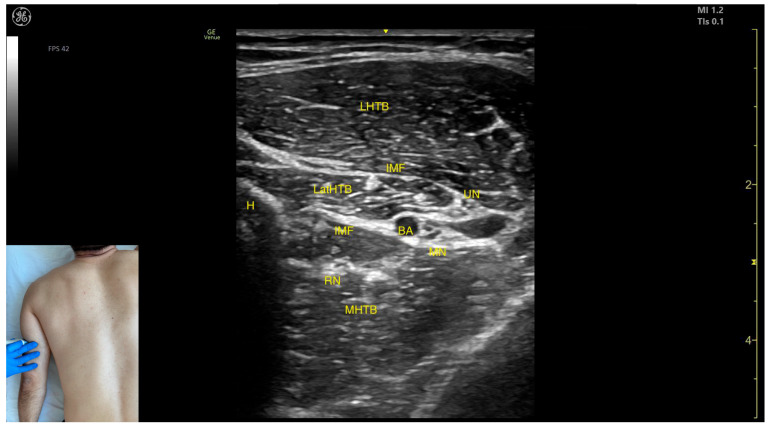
Ultrasound anatomy of the triceps brachii (TB) with key landmarks: LHTB—long head triceps brachii; IMF—intramuscular fascia; LatHTB—lateral head triceps brachii; MHTB—medial head triceps brachii; UN—ulnar nerve; RN—radial nerve; MN—median nerve; BA—brachial artery; and H—humerus.
